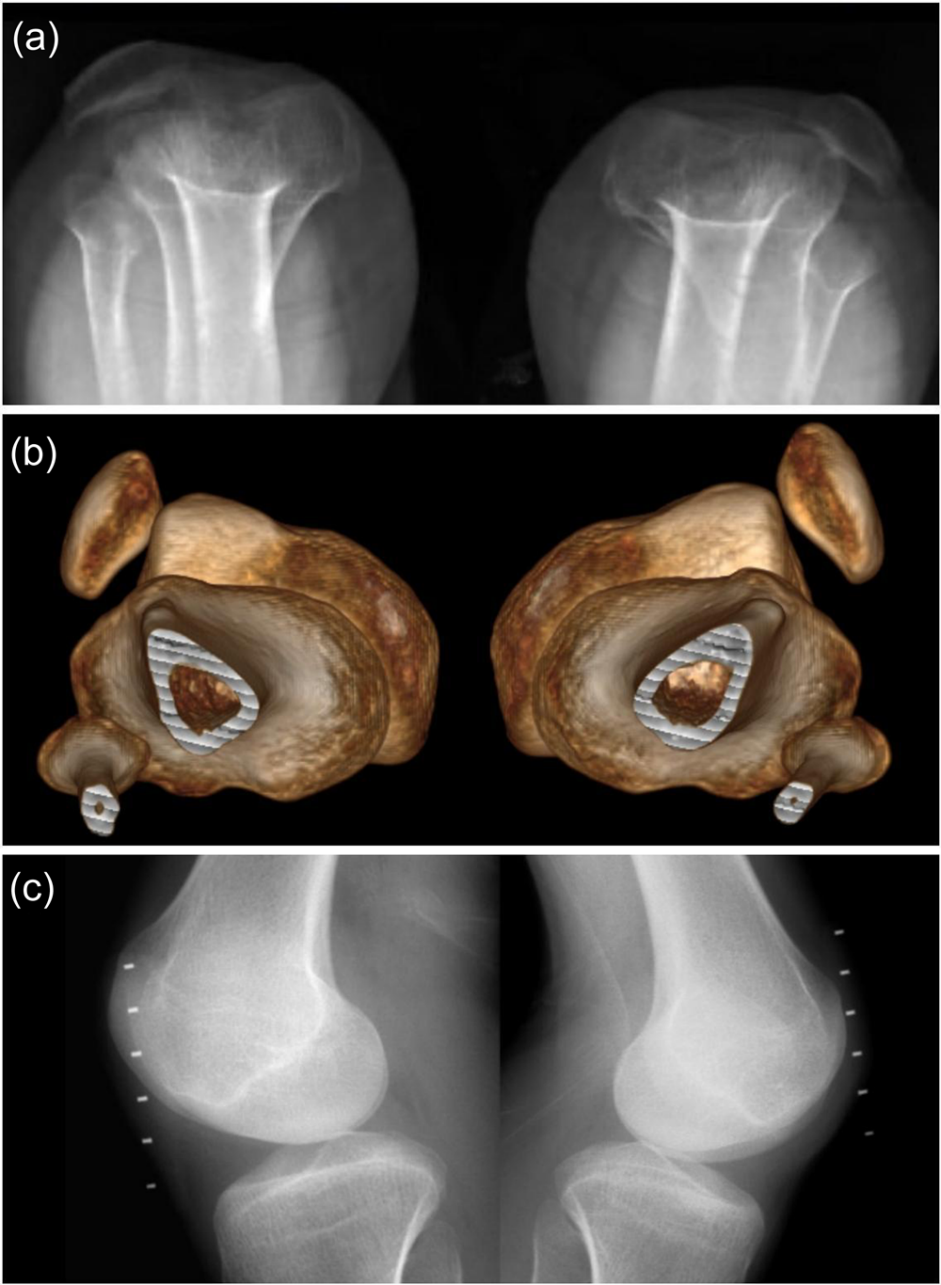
Various radiological findings associated with habitual patellar dislocation: (a) Axial views of the patellofemoral joint at maximum knee flexion angle, showing lateral dislocation of the patella. (b) Bilateral severe habitual patellar dislocation depicted in a three‐dimensional computed tomography scan, demonstrating lateral dislocation of the patella on both knees when extended. (c) ‘Invisible patella’ phenomenon, where the patella is already laterally dislocated at 30° of knee flexion. This condition prevents accurate measurement of the Caton–Deschamps index due to the patella's displaced position.
